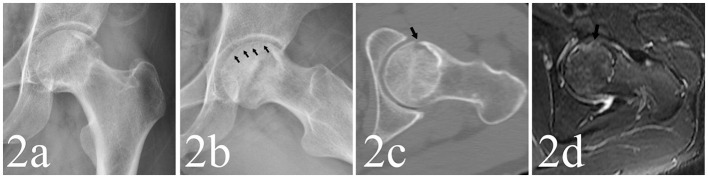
(a,b) A 33-year-old male patient whose left hip appeared as crescent sign in FL view (as the black arrows point) but not in AP view. (c,d) The CT and MRI also detected subchondral fracture in anterior part of femoral head (as the black arrows point).
